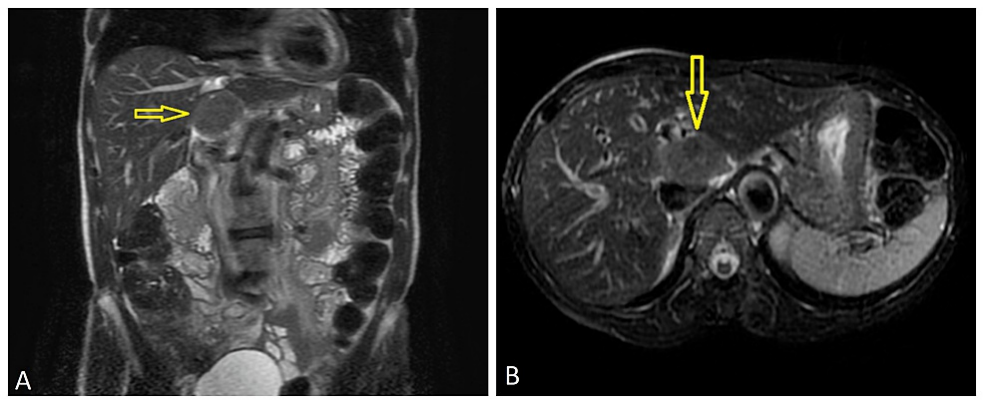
MRI abdomen with and without contrast A: Coronal view showing a 4.8 x 3.1-cm mass and numerous T1 hypointense lesions in right and left hepatic lobes. B: Axial view showing the same T2 isointense mass centered in the porta hepatis MRI: magnetic resonance imaging
